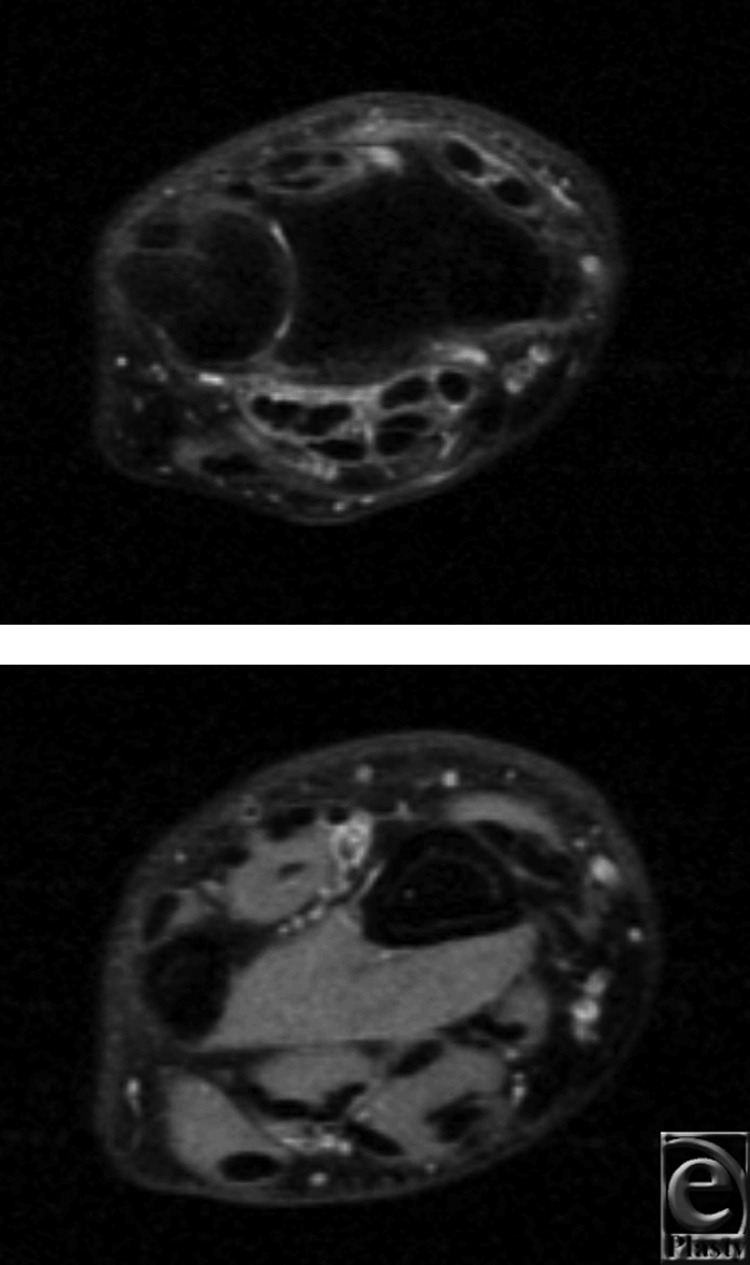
T2-weighted MRI images of patient 3 demonstrate inflammation and the absence of a tendon within the third dorsal compartment. MRI indicates magnetic resonance imaging.
